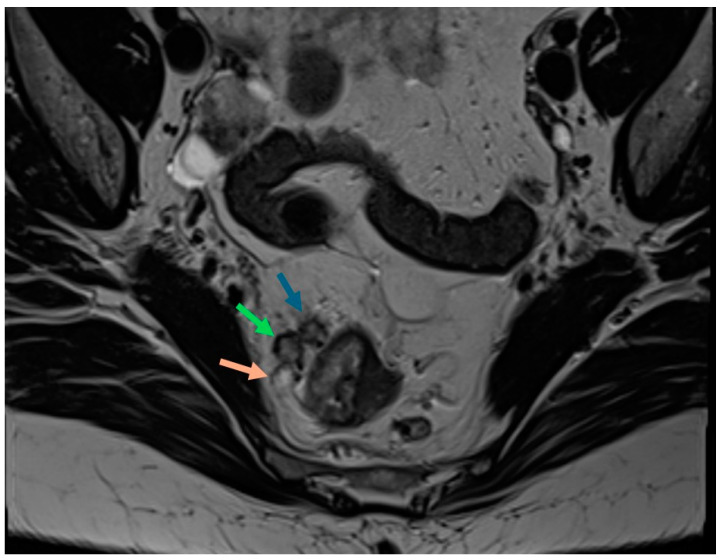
Axial T2-weighted MRI demonstrates malignant morphological features of mesorectal lymph nodes, including a round shape and heterogeneous internal signal (green arrow), irregular margins (blue arrow), and mucinous component (orange arrow).
